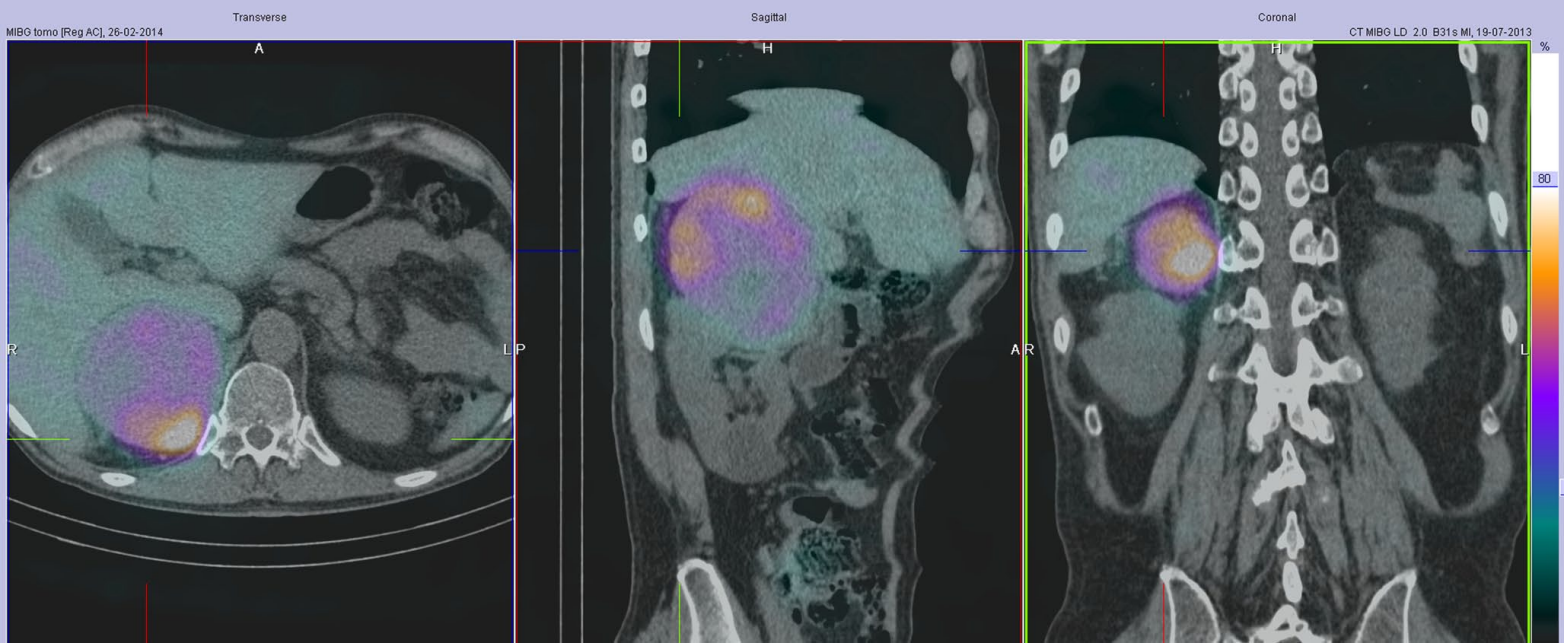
MIB SPECT scan of the thorax showing a large mass in the right upper abdomen
